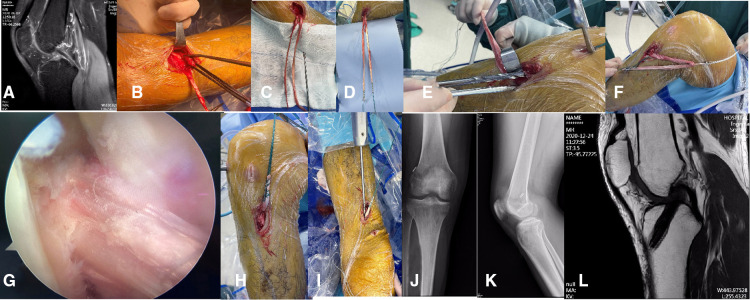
Observer patient D operation process. (A) Preoperative magnetic resonance imaging showed anterior cruciate ligament rupture. (B) The tibial insertion of the graft was preserved. (C) During the operation, tendon extractors with distal openings are used. (D) The graft is woven. (E) The stop of the graft is protected when the tibial tunnel is prepared. (F) The graft is estimated before it is placed. (G) With the help of arthroscopy, the grafts were observed to show good tension. (H,I) Interface screws were used to reinforce the graft. (J,K) Postoperative x-ray. (L) Postoperative magnetic resonance images.
